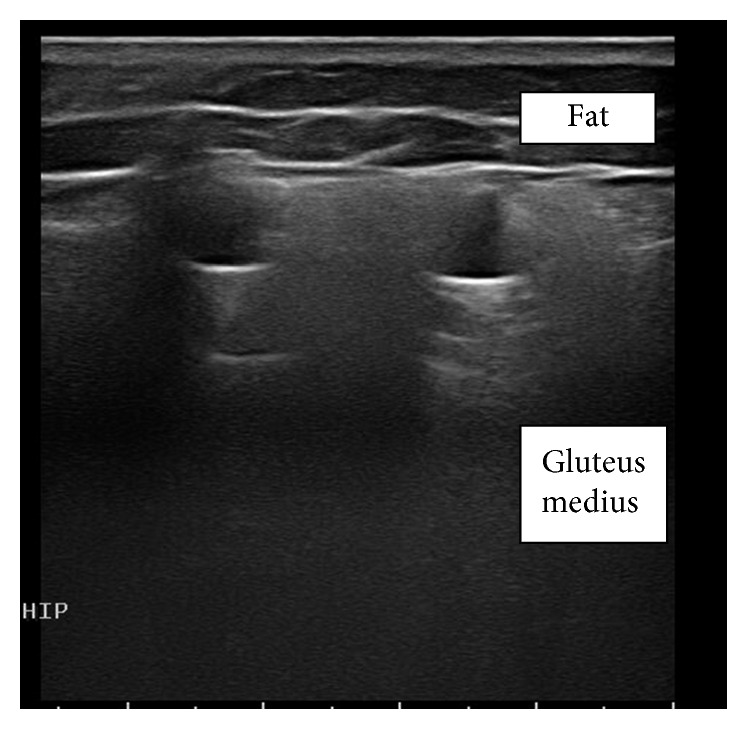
Ultrasound of the left hip showing dense hypoechogenicty of the gluteus medius with loss of normal echotexture and hyperechoic appearance as well as several anechoic areas posteriorly.
